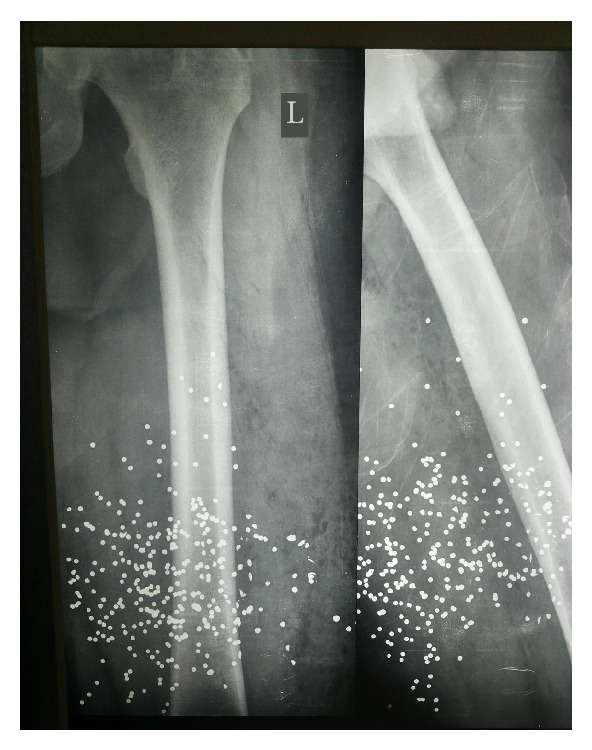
X-ray of the left femoral bone. Multiple pellets are visible in the surrounding soft tissues. No bone fracture.
